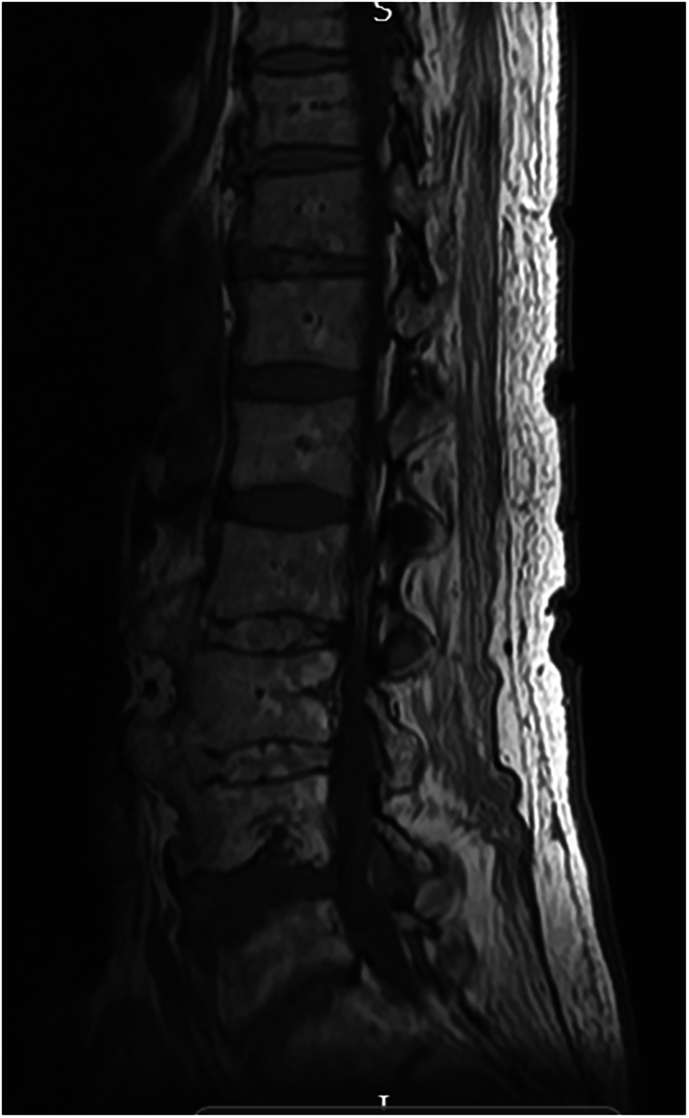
Sagittal view of the lumbar spine CT. Widening of the L4 and L5 intervertebral disc spaces with associated fluid signal and endplate irregularity, appearances may represent early Charcot’s spine.
